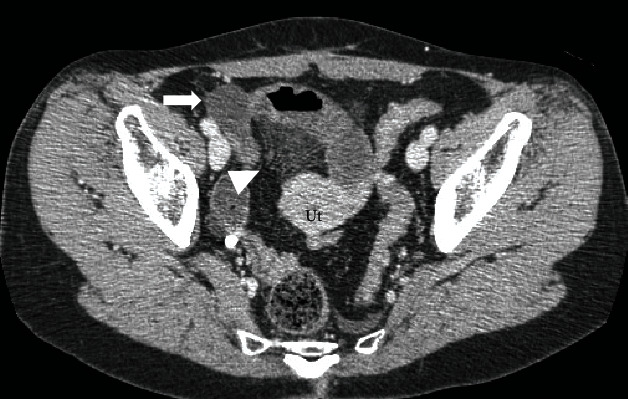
Contrast-enhanced computed tomography (CT) findings. The mesentery converges at the right side of the uterus (arrowhead). The uterus is deviated to the left. There is a spheroidal area of poor enhancement (arrow). Ut: uterus.
